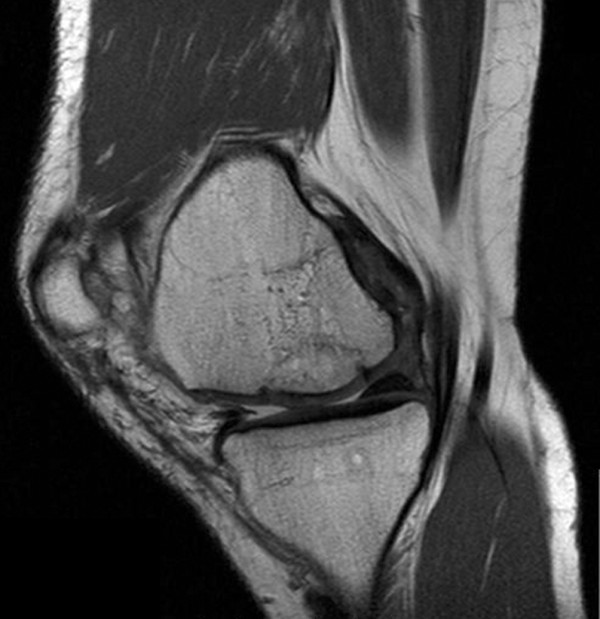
Magnetic resonance image after posterior condyle transfer. Magnetic resonance imaging scan of Patient 7, 72 months after posterior condyle transfer of the lateral femoral condyle.
